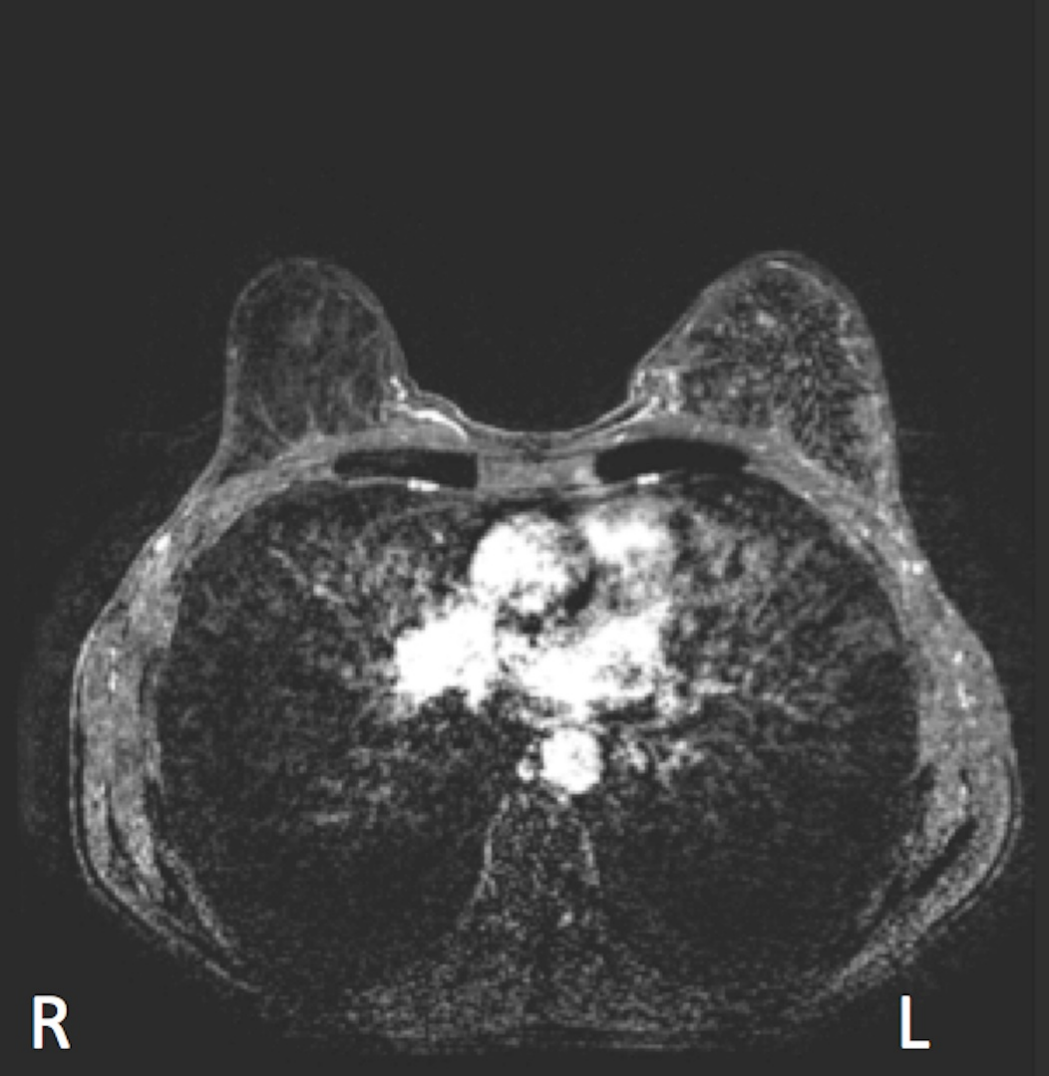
Magnetic resonance imaging showing partial response after three cycles of adriamycin/cyclophosphamide chemotherapy
